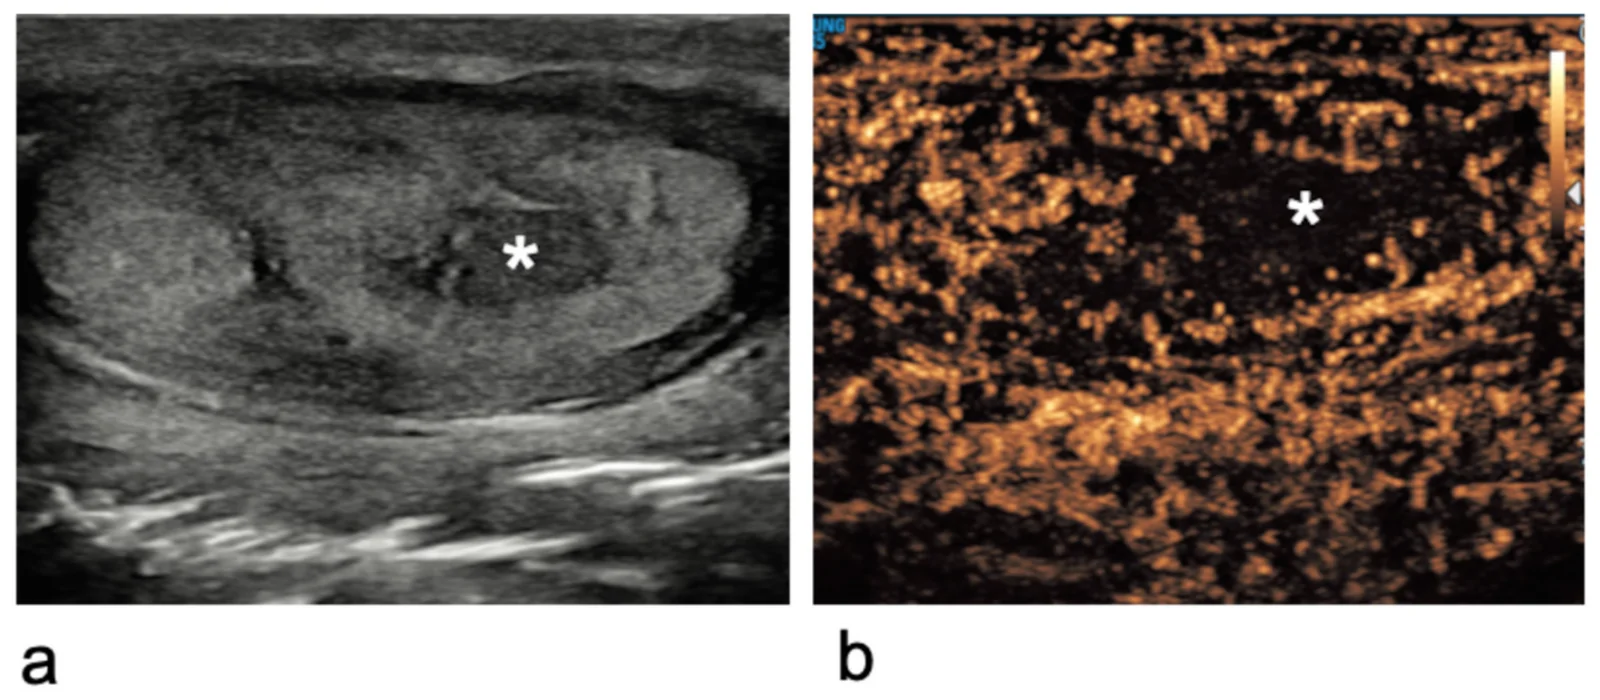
A 75-year-old man with a history of severe right epididymo-orchitis underwent ultrasonography for mild testicular discomfort. (a) Ultrasound showed a markedly heterogeneous right testis, with a focal hypoechoic lesion visible within it (asterisk). (b) CEUS demonstrated that the lesion (asterisk) was avascular, excluding an underlying neoplasm and supporting a diagnosis of post-inflammatory ischaemic changes.
